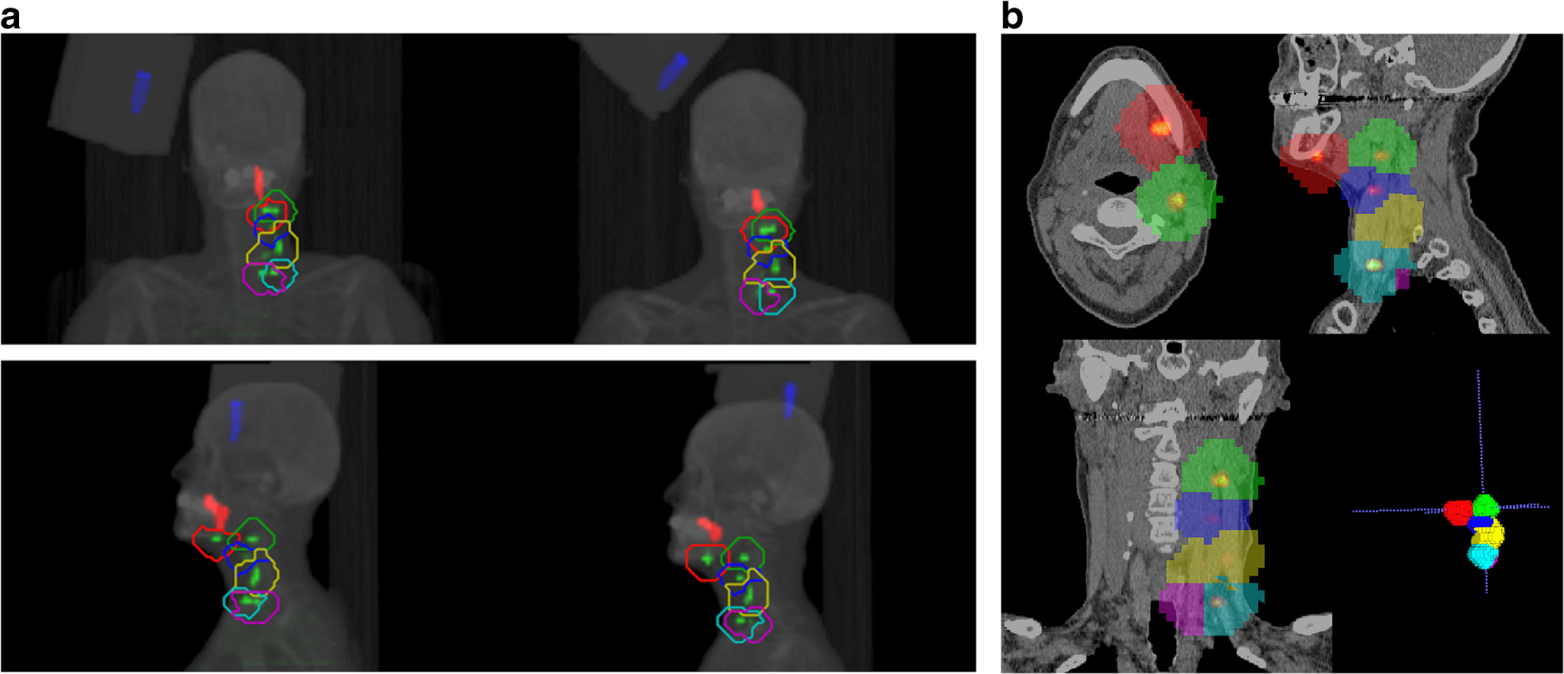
a Algorithmic-defined VOIs for all hotspots within the scanned area for both [^99m^Tc]Tc-tilmanocept and [^99m^Tc]Tc-nanocolloid. Summed intensity projections of SPECT reconstructions of the same patient, injected with either [^99m^Tc]Tc-tilmanocept (left) or [^99m^Tc]Tc-nanocolloid (right). Injection site: *Red hotspot*. Reference source: *Blue hotspot.* ‘Hot’ lymph nodes: *Green hotspots with coloured VOIs*. b Verification of VOIs containing ‘hot’ lymph nodes using 3D segmentation software (ITK-SNAP). Sentinel lymph nodes: Red and green VOI. Higher echelon nodes: blue, yellow, turquoise, and purple VOI
